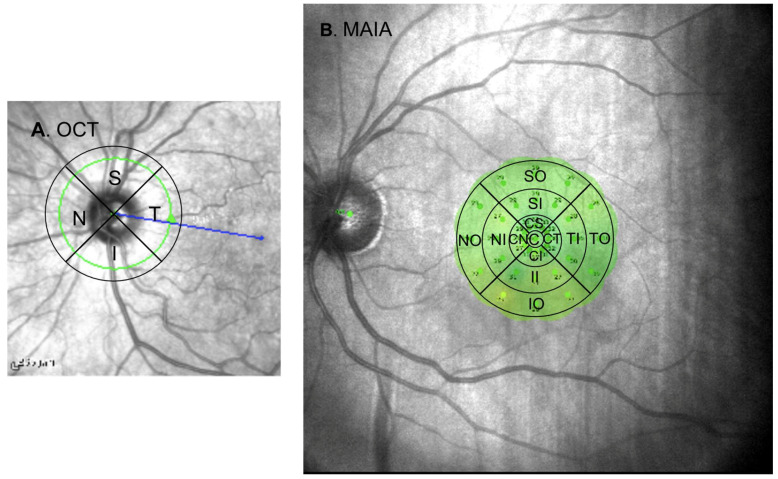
(A) Simplified representation of the OCT grid overlaid on a fundus image, with sector acronyms indicated in their corresponding regions. (B) Simplified image of the MAIA grid superimposed on a macular image as provided by the device, with the respective sector labels. Abbreviations: S: superior; T: temporal; I: inferior; N: nasal; C: central; CS: central superior; CT: central temporal; CI: central inferior; CN: central nasal; SO: superior outer; SI: superior inner; TO: temporal outer; TI: temporal inner; IO: inferior outer; II: inferior inner; NO: nasal outer; NI: nasal inner; OCT: optical coherence tomography; MAIA: macular integrity assessment.
